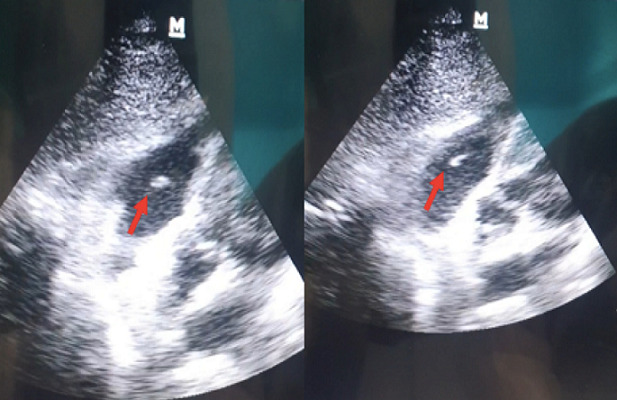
Control transthoracic echocardiography performed 2 weeks after treatment initiation showing a reduction of the tricuspid vegetation size (red arrow)
